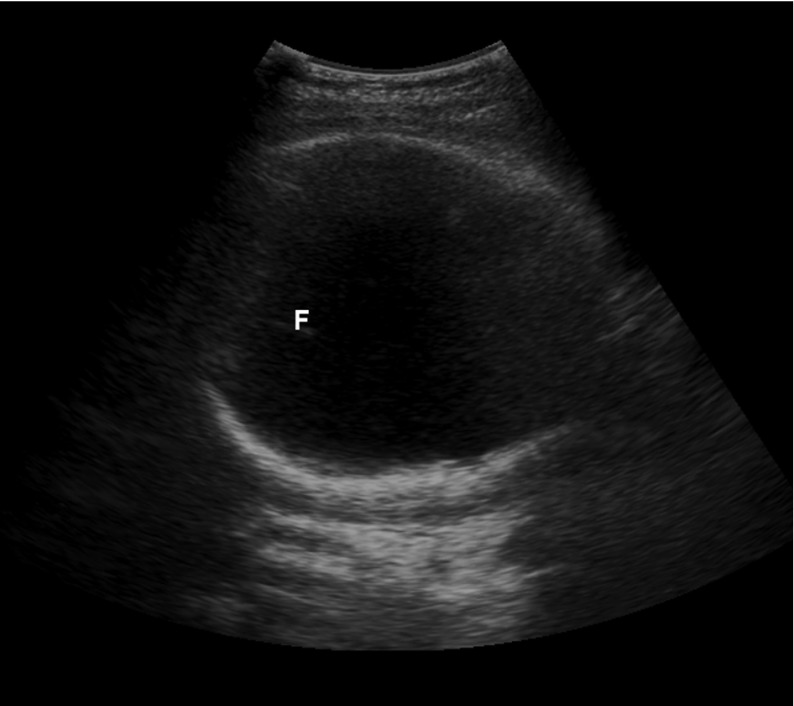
Prenatal ultrasound scans image of the fetus. Transabdominal transverse section of the fetal head shows disrupted falx (F).
